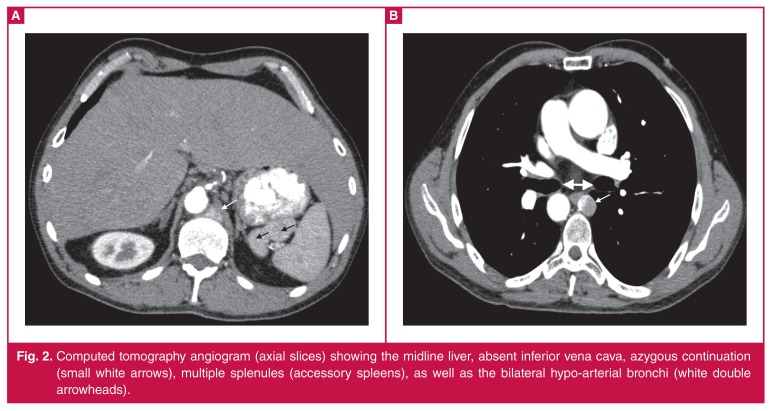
Computed tomography angiogram (axial slices) showing the midline liver, absent inferior vena cava, azygous continuation (small white arrows), multiple splenules (accessory spleens), as well as the bilateral hypo-arterial bronchi (white double arrowheads).
